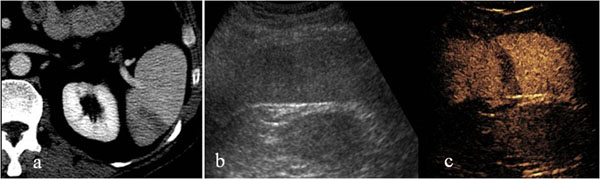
Spleen. Contrast-enhanced CT in venous phase (a), US-B-mode (b) and CEUS (c) in a 40 year- male patient with blunt abdominal trauma. CT shows a splenic hypodense parenchymal lacerative area (a) not recocognizable by US B-Mode examination (b). CEUS demonstrate splenic hypoechoic lesion corresponding to that of CT.
